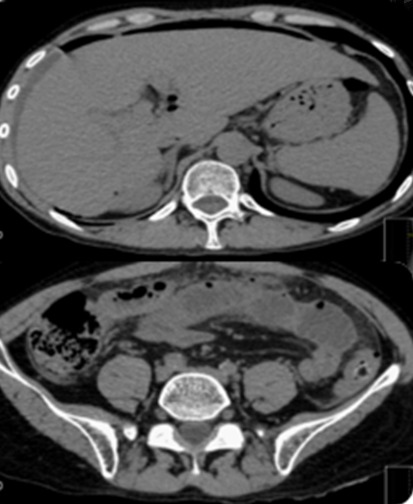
CT scan showing intra-peritoneal fluid pneumoperitoneum and bowel perforation
